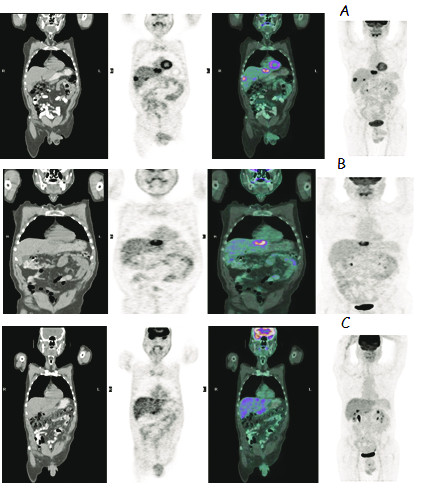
54 years-old male patient who received 1.7 GBq Y-90 microsphere therapy to the right and left lobes in separate sessions for liver metastases of colorectal cancer. 6A; coronal CT, 18F-FDG PET, fused and maximum intensity projection images of the whole body before the treatment. 6B; coronal CT, 18F-FDG PET, fused and maximum intensity projection images of the whole body after the treatment of the right lobe. 6C; coronal CT, 18F-FDG PET, fused and maximum intensity projection images of the whole body after the treatment of the left lobe.
